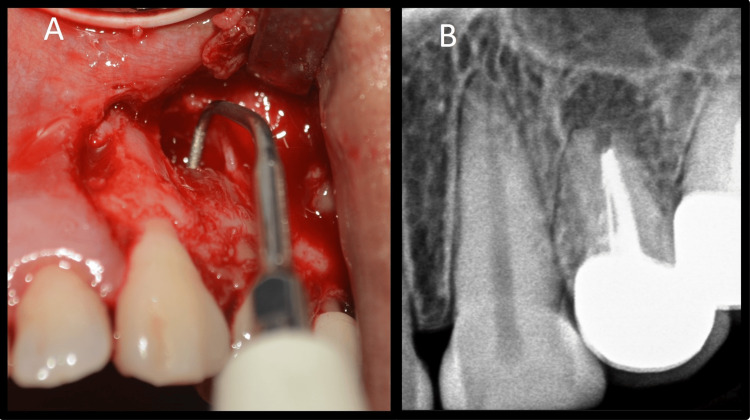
Retrograde preparation A: Root end preparation with a ultrasonic tip, B: Root end preparation radiovisiography image
